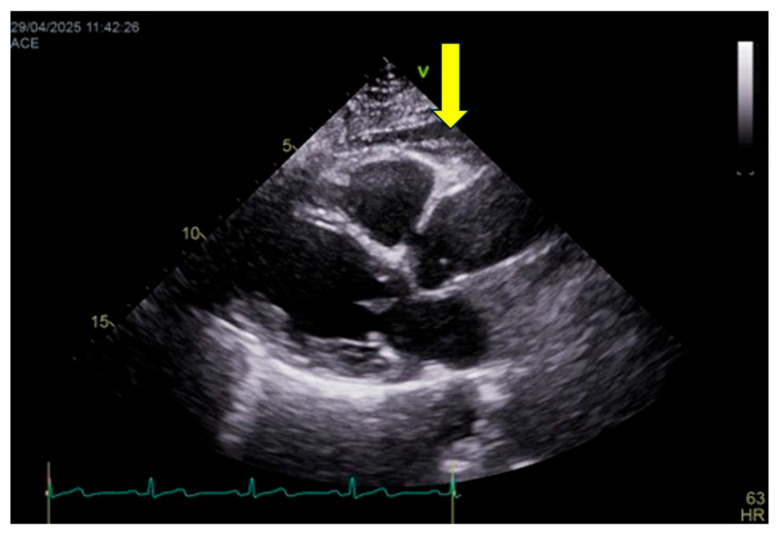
Echocardiographic Epicardial Adipose Tissue. The echocardiographic frame shows the hypoechoic adipose layer located between the visceral pericardium and the outer myocardial wall along the right ventricular free wall (arrow). EAT thickness is measured perpendicularly at end-systole, when the adipose layer is most clearly delineated.
